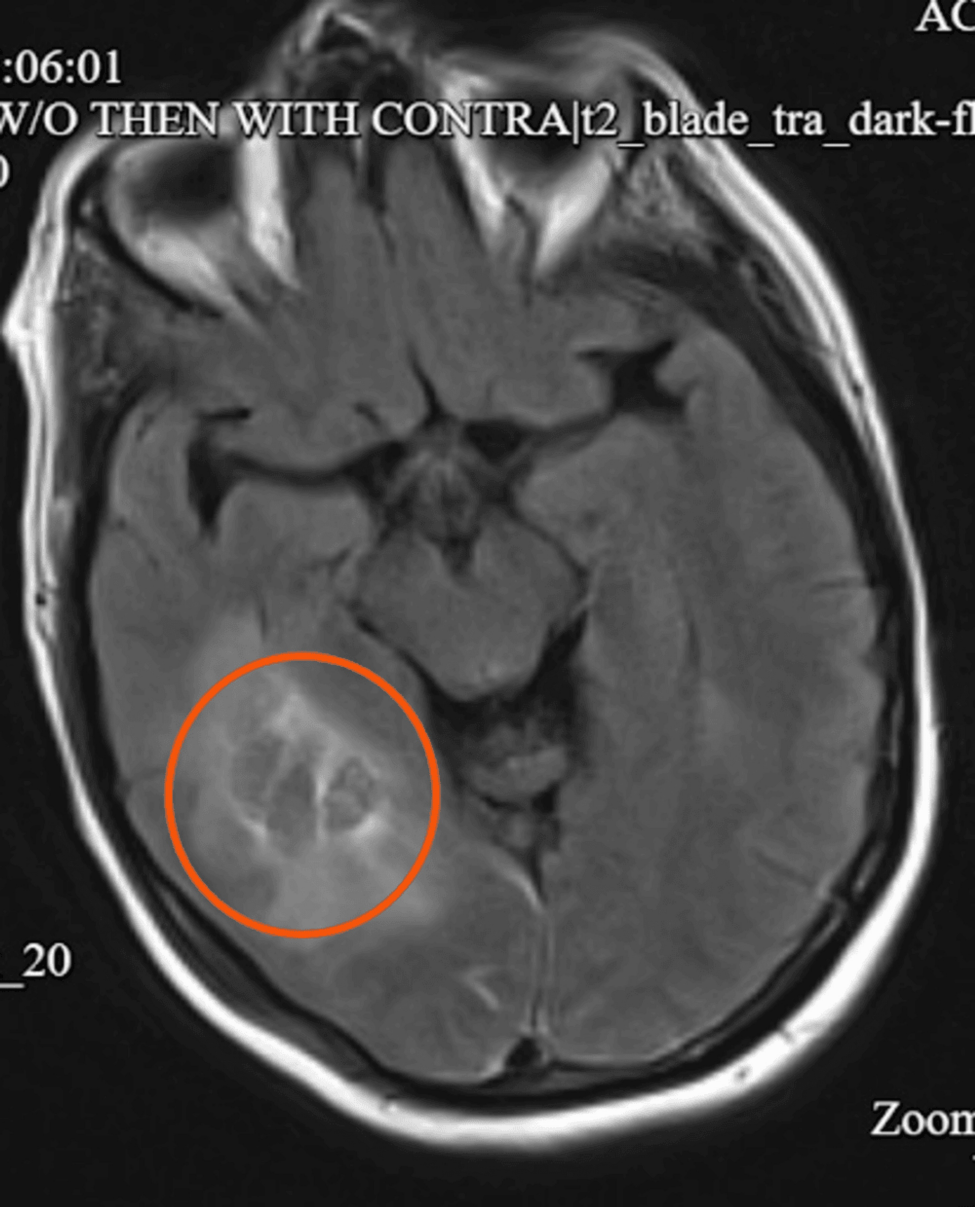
FLAIR MRI demonstrates subacute intraparenchymal hemorrhage in the right temporal lobe with significant surrounding vasogenic edema FLAIR: Fluid-Attenuated Inversion Recovery; MRI: Magnetic Resonance Imaging
